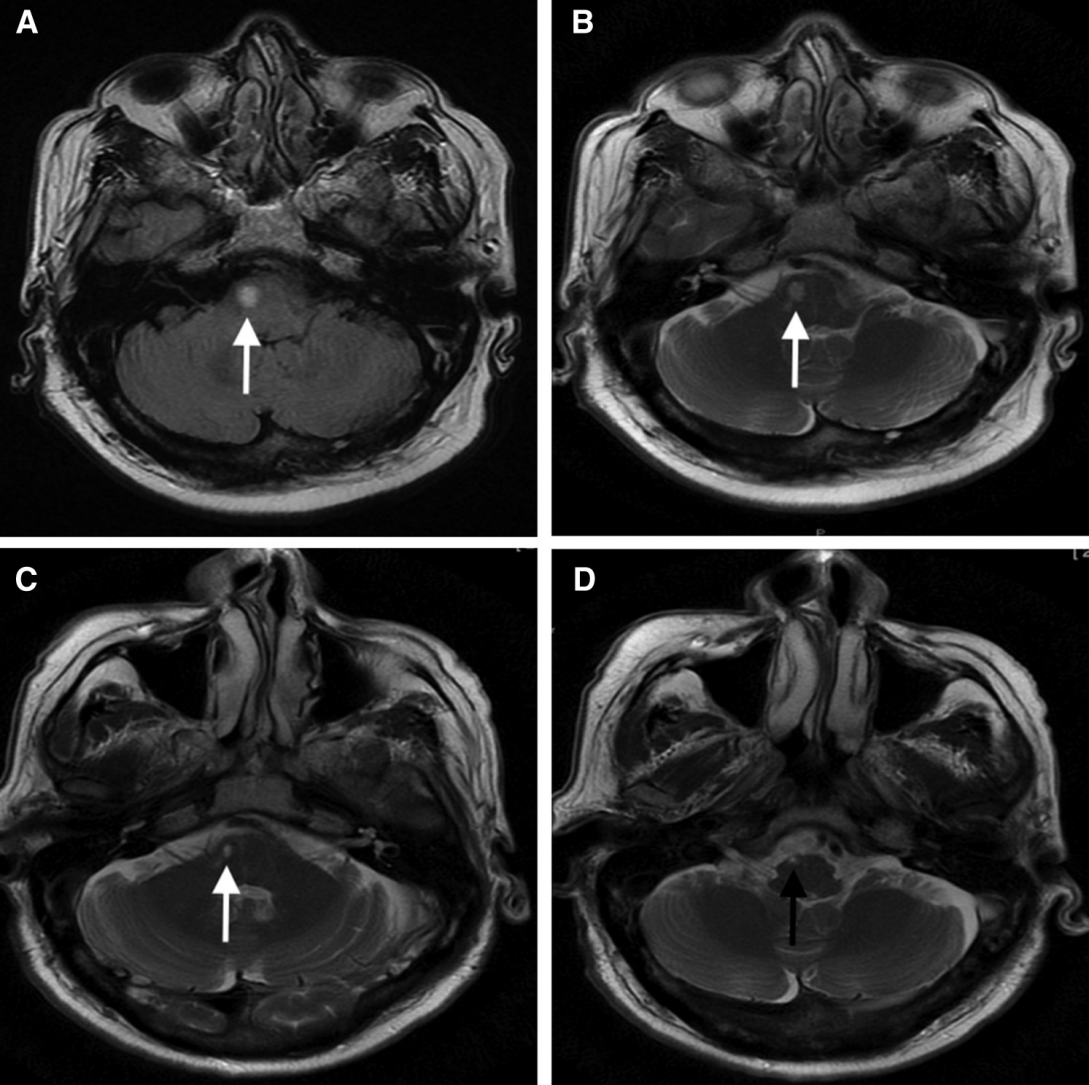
The occurrence of a white matter hyperintensity (WMH) track adjacent to index lacunar lesion. A and B, Baseline magnetic resonance imaging (MRI), performed 11 d after stroke onset, sporadic symptomatic lacunar infarct in the right pons (white arrow; fluid-attenuated inversion recovery; T2-weighted imaging). C, Follow-up MRI at 1 y (412 d) after index stroke, cavitated lacunar lesion in the right pons on T2-weighted imaging (white arrow). D, Follow-up MRI, WMH track proximal to index lacunar lesion in the right pons, visible on >2 MRI slices inferior to the index lacunar lesion (black arrow; T2-weighted imaging).
